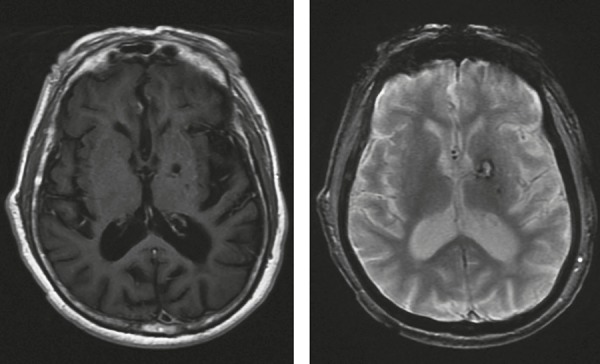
T1 and T2* magnetic resonance imaging sequences depicting old infarct
